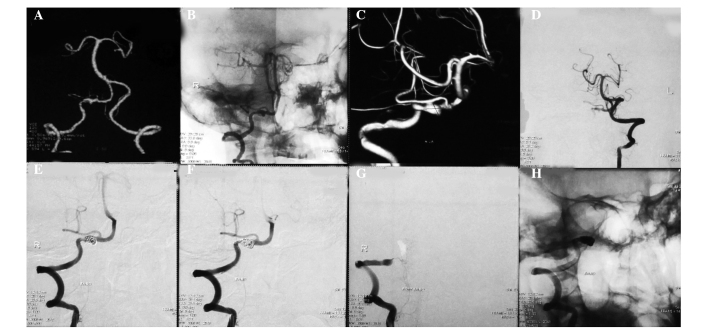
(A) Computed tomography angiography image showing the bilateral vertebral artery. (B) Negative and (C) 3D digital subtraction angiography (DSA) images showing a well-developed type IIIa dissecting aneurysm of the right vertebral artery located proximally to the posterior inferior cerebellar artery and the contralateral vertebral artery. The aneurysm was treated with coiling combined with right vertebral artery occlusion. (D) DSA image of the left vertebral artery. The post-treatment angiograms show (E) complete obliteration of the aneurysm and (F and G) occlusion of the distal part of the right vertebral artery. (H) Negative image of the right vertebral artery following treatment.
